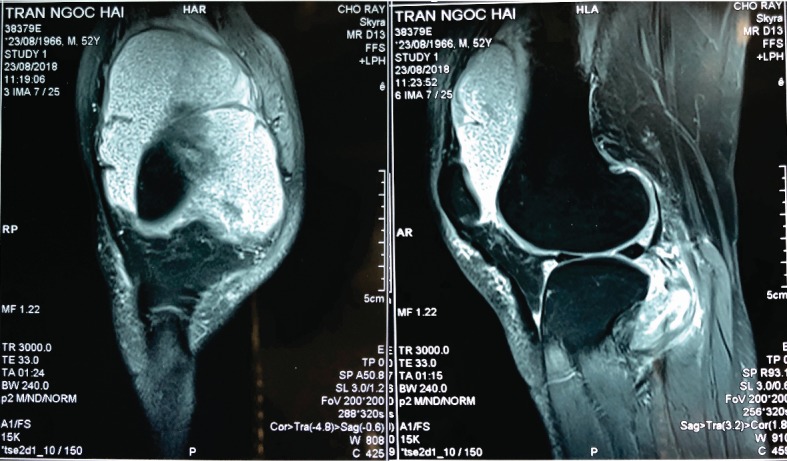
MRI showed multiple rice bodies.
